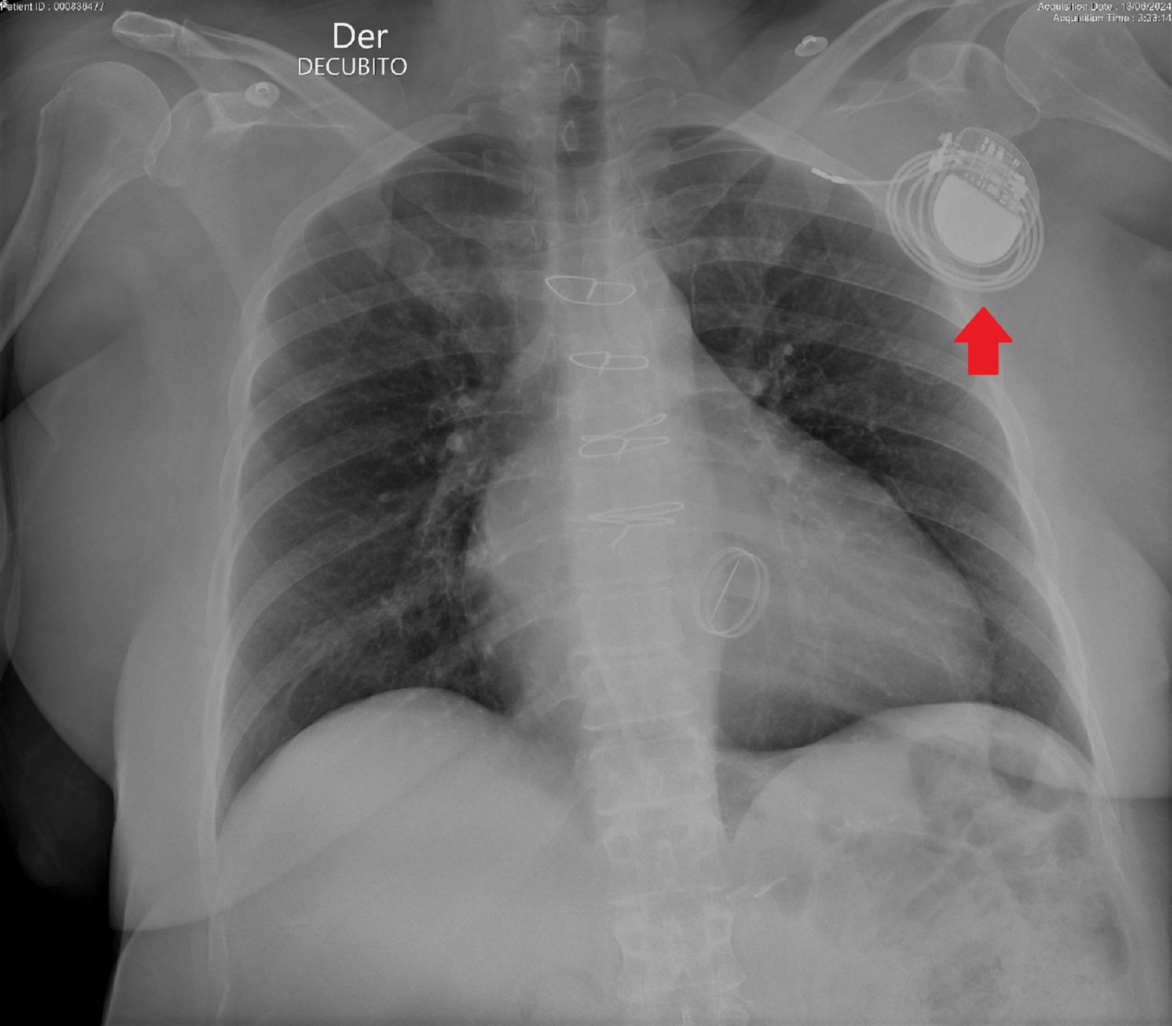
Chest X-ray showing ventricular electrode displacement and twisted lead near the generator
